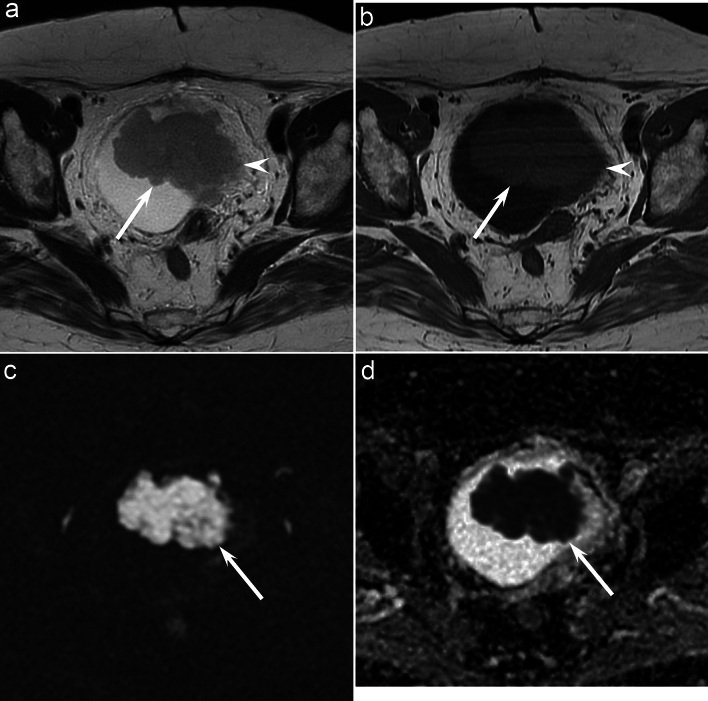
A 76-year-old woman with bladder SCNEC. Axial T2-weighted image a and Axial T1-weighted image b indicate a broad-based bladder mass (arrow) with a smooth lobulated margin and extension into perivesical fat (arrowhead). c Axial diffusion-weighted image shows a mass that is markedly hyperintense (arrow). d Axial ADC map shows markedly restricted diffusion with decreased ADC values (0.533 × 10^−3^ mm^2^/s) (arrow)
